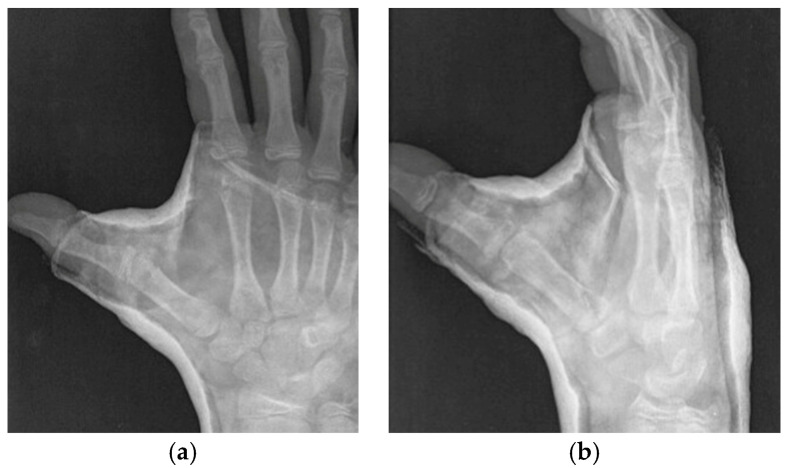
X-ray views of TMC joint after closed reduction. (a) AP view. (b) Lateral view.
